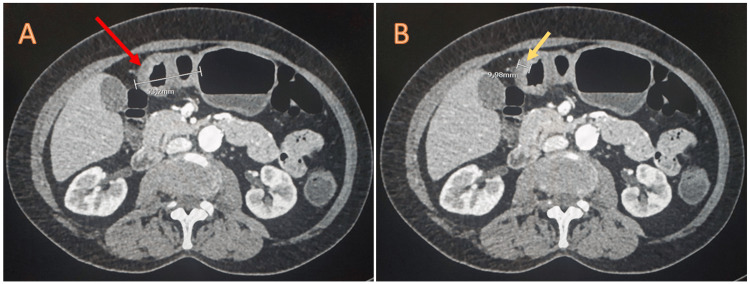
Contrast-enhanced abdominal CT (axial view) showing the gastric lesion. (A) The lesion measuring 5.3 cm. (B) Wall thickening measuring 10 mm.
